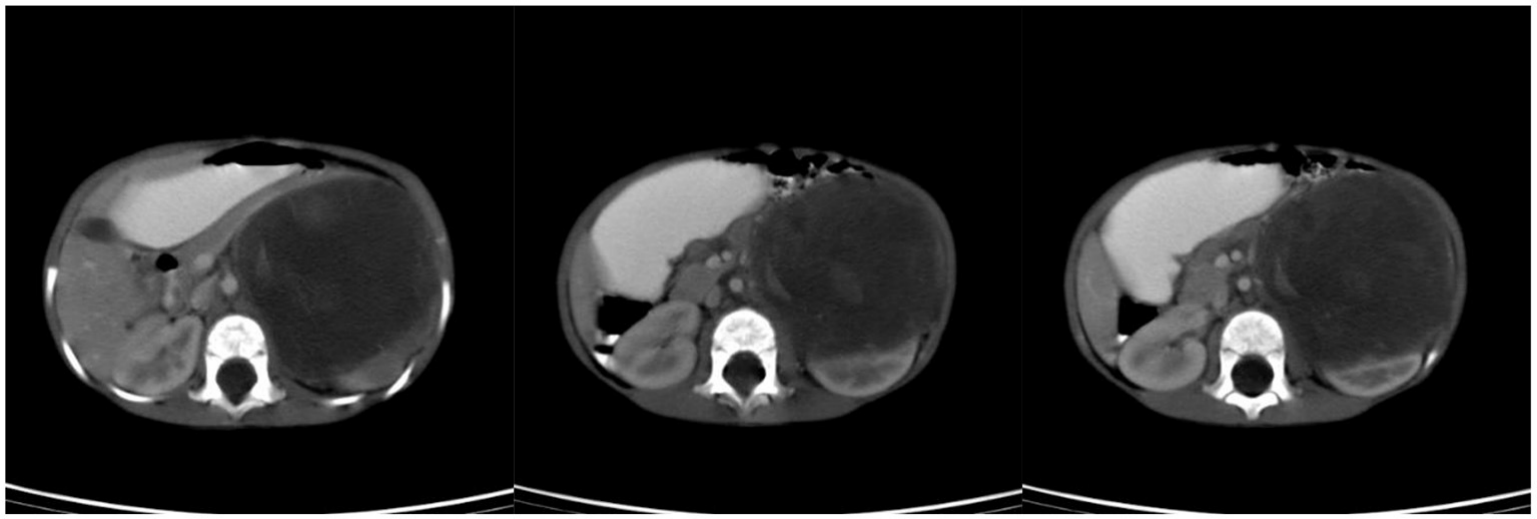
Preoperative CT scan of patient C.
